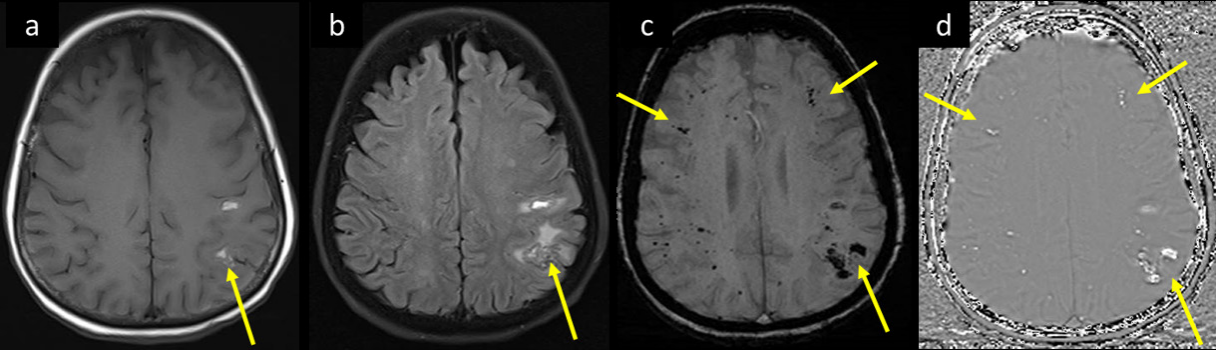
A 66-year-old female with known DM, and HTN, admitted with COVID pneumonia, progressed to severe ARDS requiring intubation, had deterioration of the level of consciousness, acute renal failure, and seizures with jerky movements of right upper limb. MRI brain axial images at supraventricular level (a) *T*_1_WI, and (b) *T*_2_-FLAIR shows pre- and post-central cortical/juxtacortical microbleeds with bright signal in *T*_1_ and FLAIR, and surrounding parenchymal edema (arrows in a, b). SWI at the same level (c) magnitude and (d) phase axial images demonstrates corresponding dephasing signal/blooming, as well as widespread punctate microhemorrhagic changes (long and short arrows respectively in c and d). ARDS, acute respiratorydistress syndrome; DM, diabetes mellitus; FLAIR, fluid attenuated inversionrecovery; HTN, hypertension; SWI, susceptibility-weighted imaging
